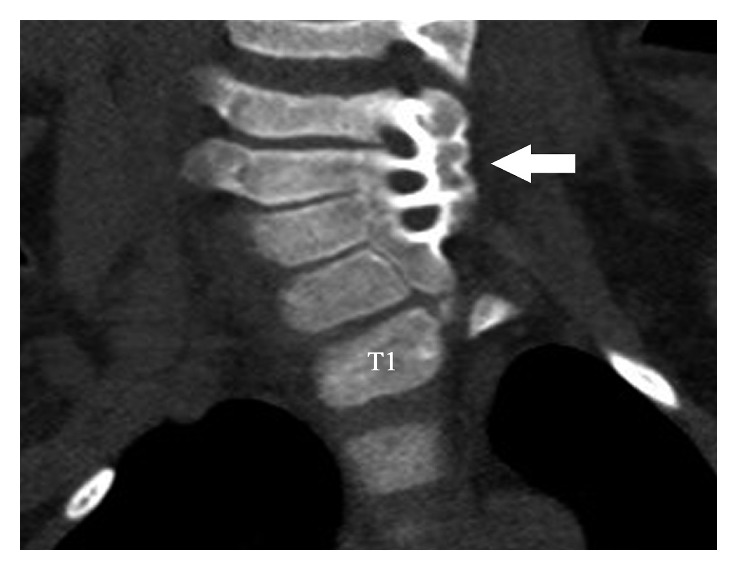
CT, coronal reformatted image demonstrates partial fusion involving the left cervicothoracic spine from C4 through T1 in a patient with Klippel–Feil syndrome (arrow). (*Courtesy of Miguel Flores*, *MD*, *Orlando*, *FL.*)
